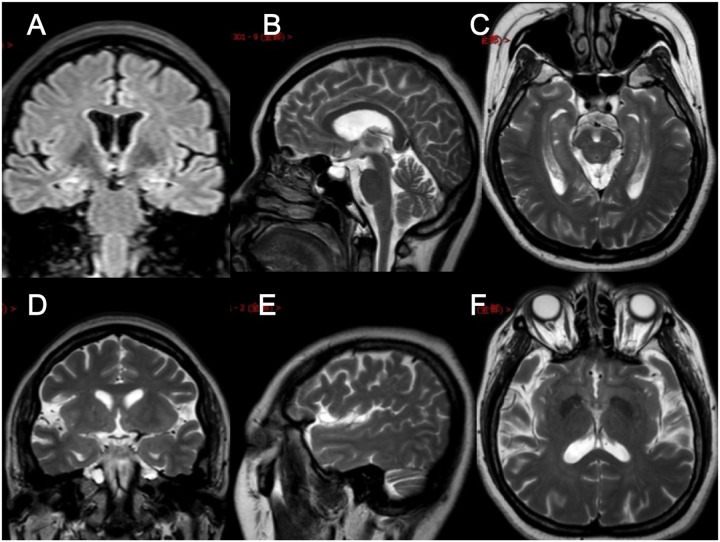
Brain MRI images of the proband. (A–F) Brain MRI images of different levels of the proband show hippocampal atrophy. (A) FLAIR in the coronal plane; (B,E) T2WI in the sagittal plane; (C,F) T2WI in the axial plane; (D) T2WI in the coronal plane.
